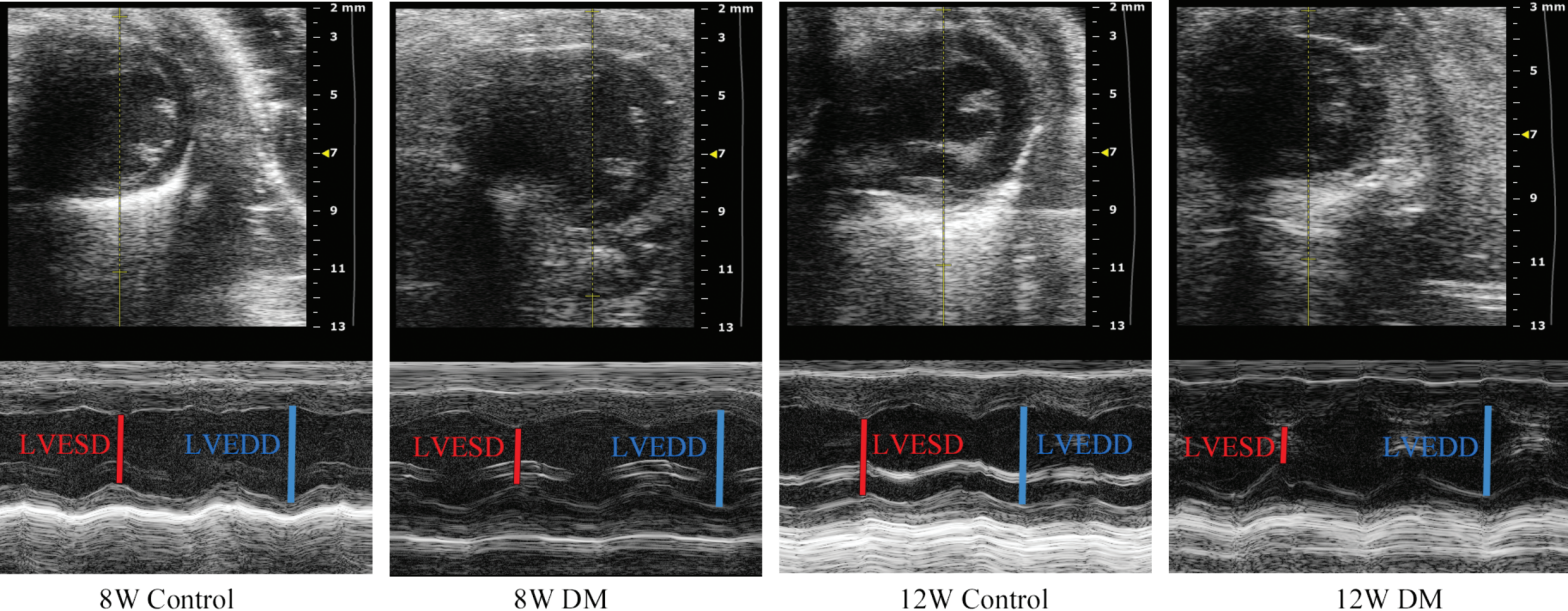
Echocardiographic images demonstrating an example of left ventricular diameter in M‐mode. LVESD, Left ventricular end‐systolic diameters; LVEDD, Left ventricular end‐diastolic diameters
